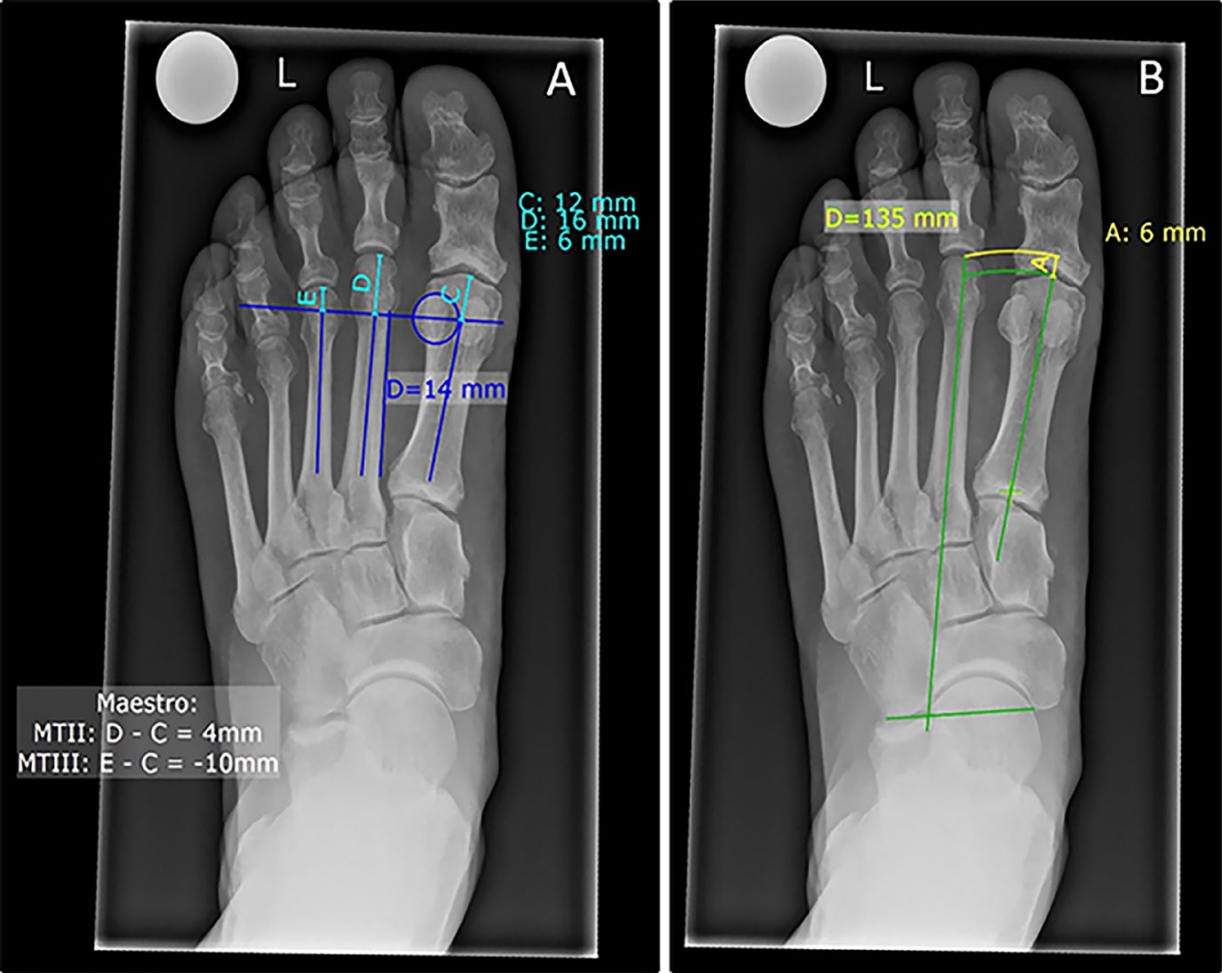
Radiographs of the left foot (dorsoplantar view, weightbearing) of a patient who is to undergo first-ray amputation. (A) Visualization of the Maestro technique marked with blue lines and measured in turquoise (“C, D, E”). (B) Hardy-Clapham technique marked with green lines and measured in yellow (“A”). [See online article for color figure.]
